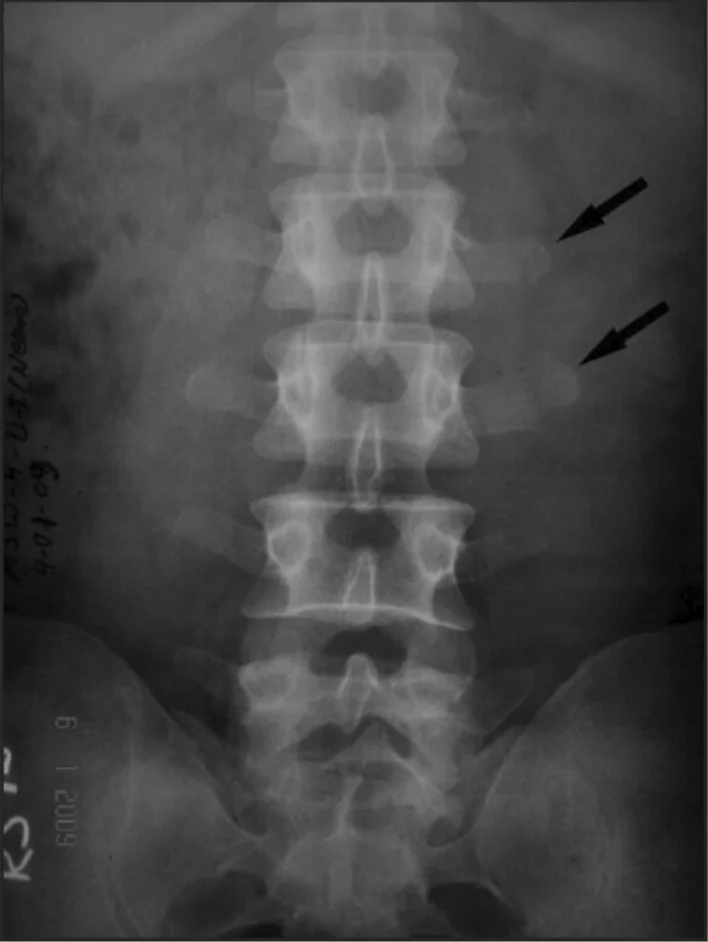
Plain film radiograph of the lumbar spine showing fractures of the left L2 and L3 TPs [22]; reproduced from Agrawal et al. [22], with permission
